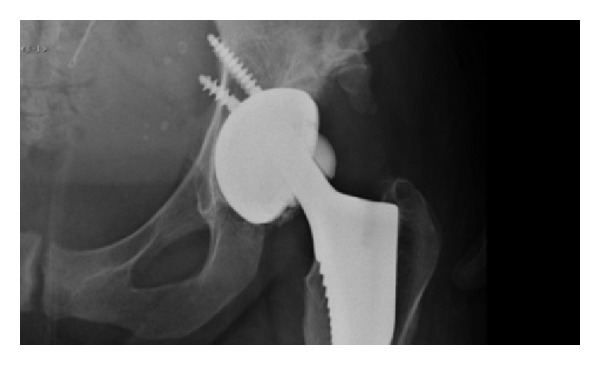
Radiograph demonstrating subtle fragmentation at the inferior head/neck junction.
